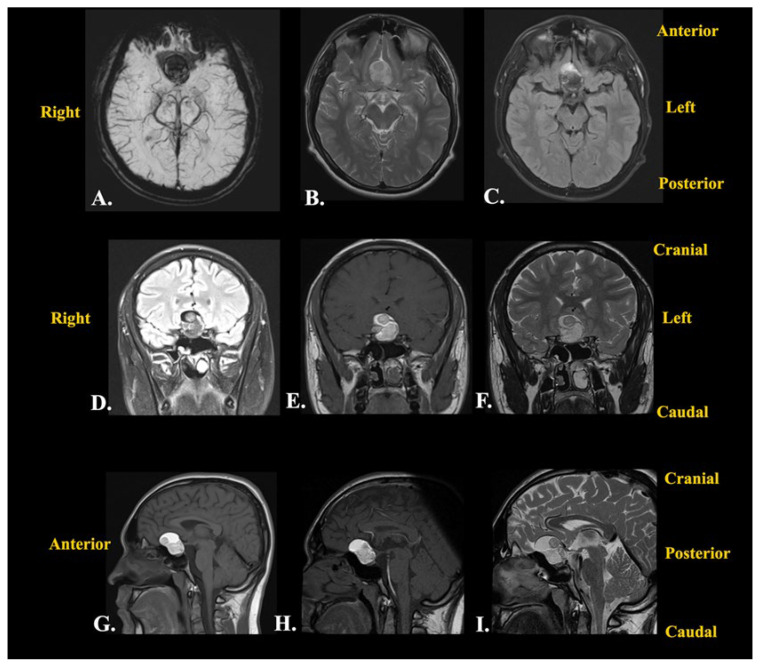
Preoperative contrast-enhanced brain MRI performed before the patient’s admission into our department: (A) axial susceptibility-weighted imaging sequence; (B) axial T2-weighted imaging Fast or Turbo Spin Echo (FSE/TSE) sequence; (C) axial Transverse Relaxation Time (T2)-weighted imaging TSE sequence using dark fluid; (D) coronal T2-weighted dark fluid sequence; (E) coronal T1-weighted dynamic contrast-enhanced sequence; (F) coronal T2-weighted TSE sequence; (G) sagittal T1-weighted TSE sequence; (H) sagittal T1-weighted contrast-enhanced sequence; (I) sagittal T2-weighted TSE sequence.
